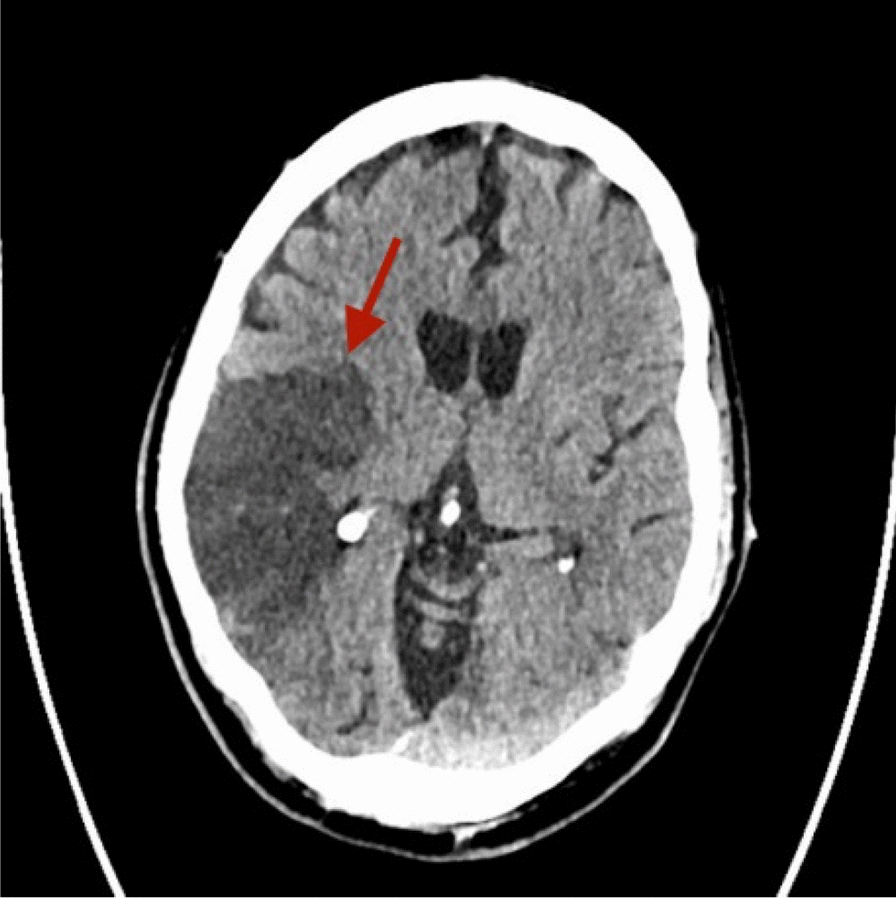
Non-contrast CT scan of right middle cerebral artery (MCA) territory (temporoparietal region). [42] Copyright © 2024, Rashwan et al.
